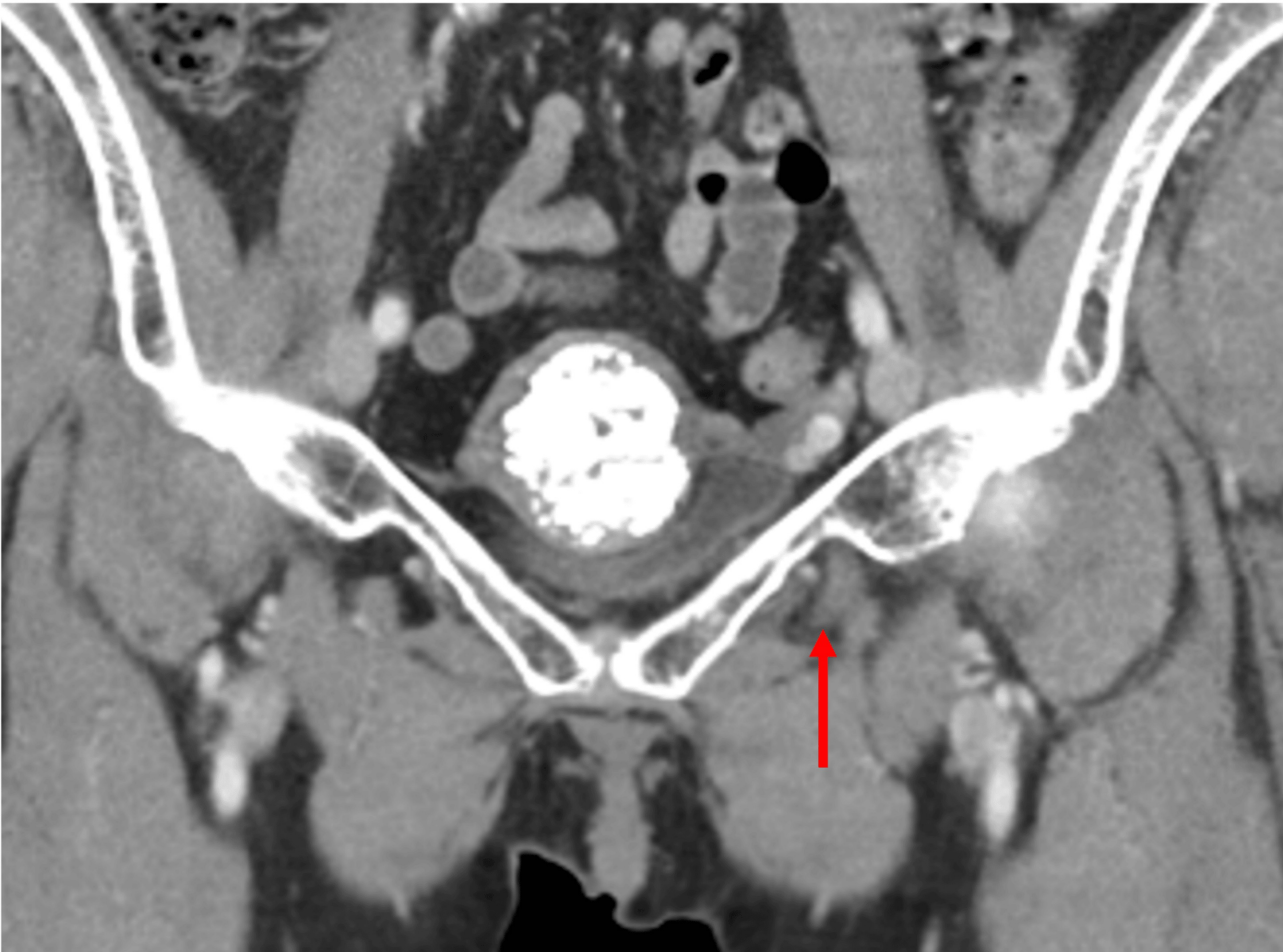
Contrast-enhanced coronal computed tomography image showing the distal extent of the decompressed bladder diverticulum as it passes through and terminates within the obturator foramen.
